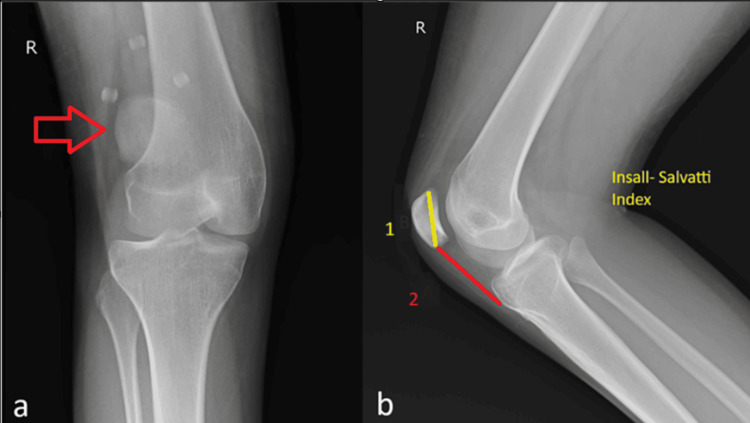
Radiography of the right knee (a) Anteroposterior view with arrow showing patella displaced laterally. (b) Lateral view showing Insall-Salvati Ratio > 1.5 indicative of patella alta, where point 1 represents the length of the patella and point 2 represents the length of the patella tendon
